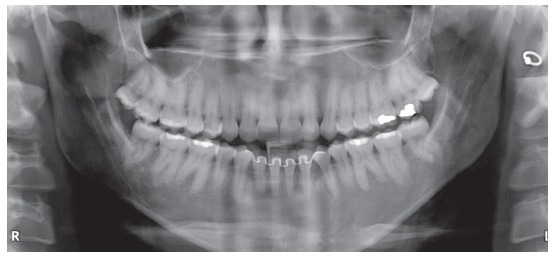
Seven-years posttreatment panoramic radiograph.
